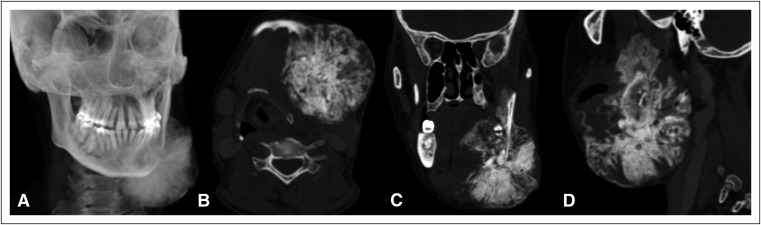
(A) Reconstructed radiograph and (B, C, and D) computed tomography images (B: axial; C: coronal; and D: sagittal in bone algorithm) reveal significant increases in the extent and size of the mass, particularly the osseous periosteal reaction.
